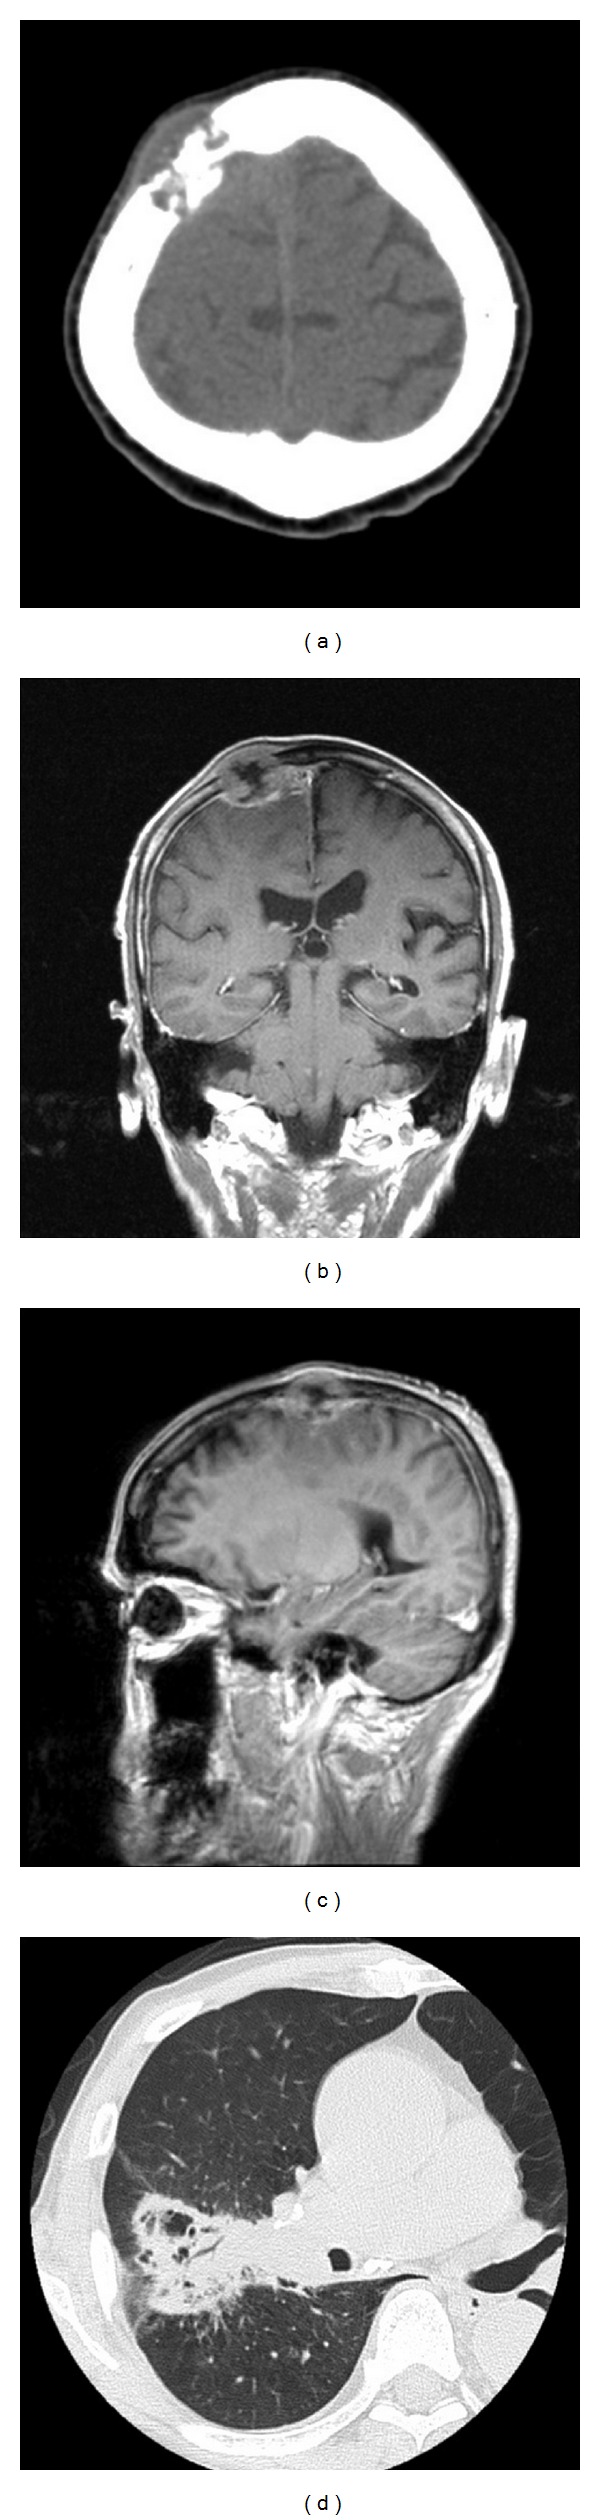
Computed tomographic scan of the brain shows an osteolytic tumor in the right frontal region (a). Coronal (b) and sagittal (c) T1-weighted magnetic resonance images with gadolinium-diethylenetriaminepenta-acetic acid revealing a heterogeneously enhanced tumor in the right frontal region with perifocal edema. The tumor was attached to the wall of the superior sagittal sinus and dura. Computed tomographic scan of the chest reveals an endobronchial growth in the hilum of the right upper lobe (d).
